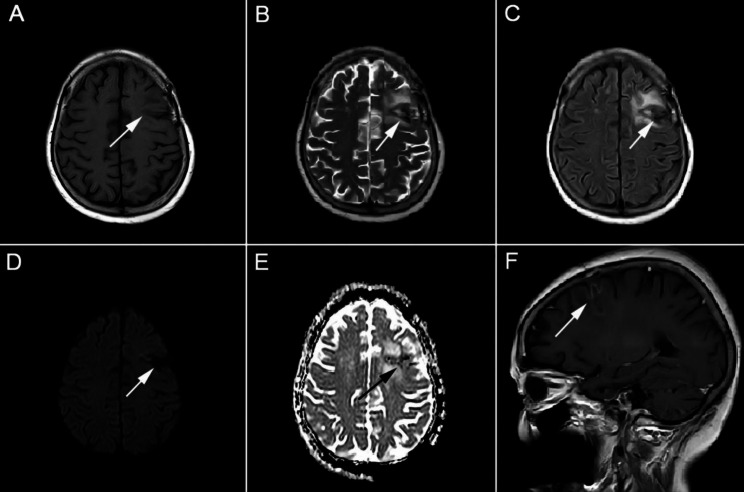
1.5-T MRI of brain at the 5 months follow up. Abnormal hypointensity on T1-weighted images (A), hyperintensity on T2-weighted images (B), and hypointensity on fluid-attenuation inversion recovery (FLAIR) images are observed in the brain lesions’ core (C). Additionally, hyperintensity around the brain lesions’ core on FLAIR imaging (D) suggested the softening of the lesions and secondary gliosis, as seen on coronal MRI slices. Hyperintensity on diffusion-weighted imaging (DWI) in the brain lesions disappeared (D) and hyperintensity on apparent diffusion coefficient (ADC) imaging increased (E), as seen on coronal MRI slices. Brain abscesses showed slight enhancement, as seen on the MRI sagittal image (F). White and black arrows indicate lesions
